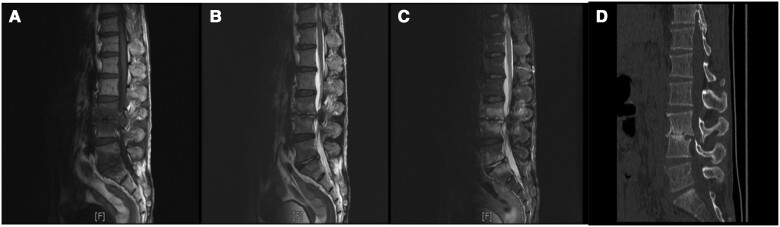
Magnetic resonance (MR) imaging and computed tomographic (CT) results in a 57-year-old man with spinal brucellosis. *A–C,* Sagittal T1-weighted (*A*) and T2-weighted (*B*) and T2-weighted fat-suppression (*C*) MR images show multiple abnormal signals in L3, L4, L5, and S1 vertebral body levels. *D,* CT sagittal reconstruction indicated L3 and L4 vertebral body destruction and intervertebral space narrowing.
